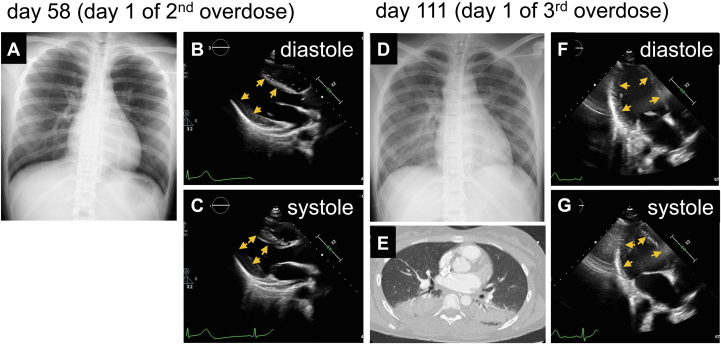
Chest Radiography, Computed Tomography, and Echocardiogram Performed on Days 58 and 111 (A) Chest radiography and (B, C) echocardiography revealed nearly normal findings. (D) Chest radiography and (E) CT revealed pulmonary congestion and dorsal consolidation. (F, G) Echocardiography demonstrated the pattern of reverse Takotsubo cardiomyopathy. Yellow arrows indicate the endomyocardial surface.
